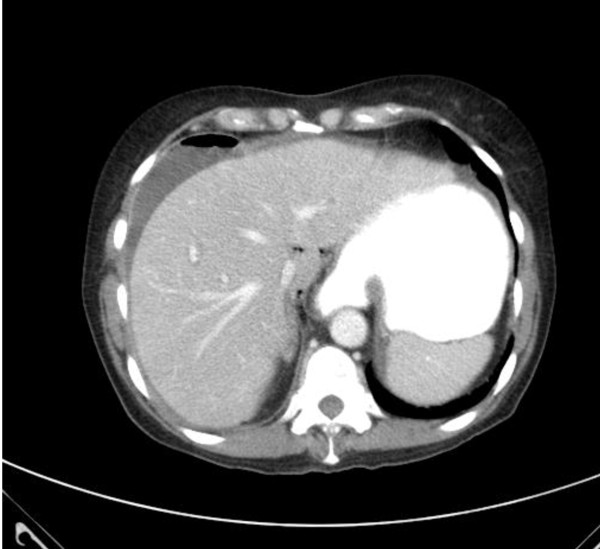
A computed tomography (CT) scan of the abdomen and pelvis showing free intra-peritoneal air consistent with a perforated viscus.
